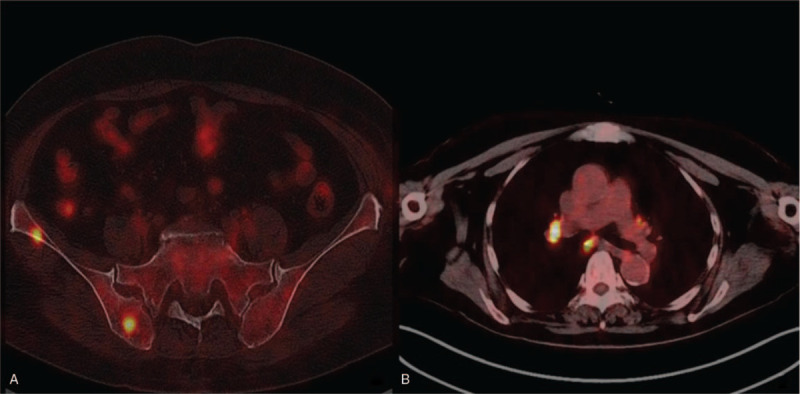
PET-CT. Multiple metastases in the lymph nodes and bones. A: bone metastasis; B: lymph node metastasis.
